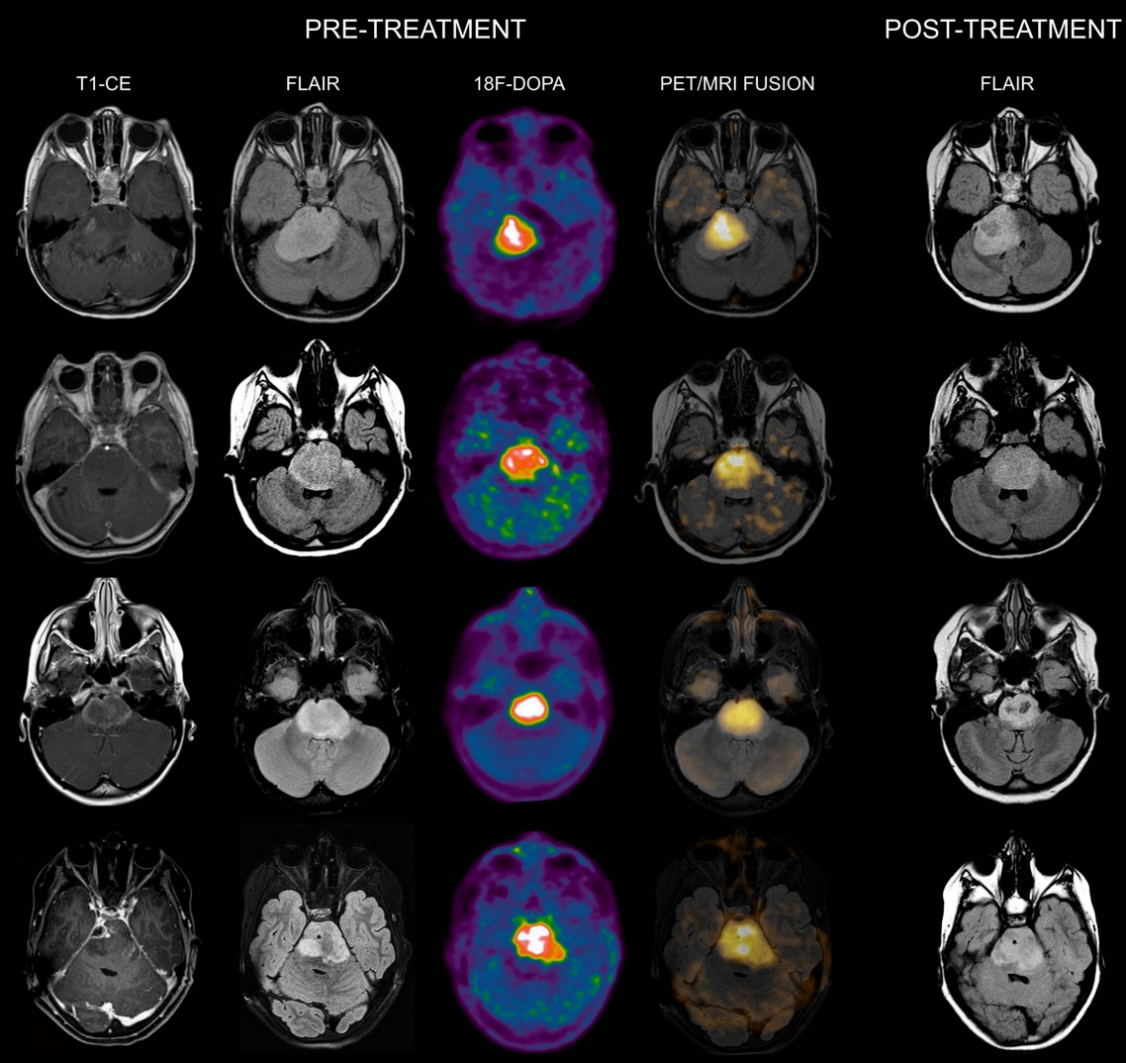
Co-registered and fused MRI and ^18^F-DOPA PET images of DIPGs with T/S > 1. First row: 4-year-old female. Pre-treatment contrast-enhanced (CE) T1-weighted imaging showed ring enhancement. MRI tumor volume was 40 cc. ^18^F-DOPA PET and fused ^18^F-DOPA PET/MRI revealed markedly increased uptake (T/S 1.09) with a PET tumor volume of 21 cc, corresponding to a uniformity of 52.5%. Post-treatment FLAIR demonstrated an MRI tumor volume of 28.9 cc. Overall survival (OS) was 9 months. Notice the coincidence between the tumoral component with increased uptake and the residual lesion, and the tumor volume reduction in the left ventrolateral pons corresponding to absence of increased uptake. Second row: 7-year-old male (anaplastic astrocytoma, H3K27M-mutant). Pre-treatment CE T1-weighted imaging did not reveal areas of ring enhancement. MRI tumor volume was 34.06 cc. On ^18^F-DOPA PET and fused ^18^F-DOPA PET/MRI the T/S was 1.27, the tumor volume 25 cc, and the uniformity 73.39%. Following treatment, the MRI tumor volume was of 33.7 cc. OS was 10 months. Third row: 10-year-old female. On pre-treatment CE T1-weighted imaging there was extensive ring enhancement. MRI tumor volume was 26 cc. On ^18^F-DOPA PET and fused ^18^F-DOPA PET/MRI the lesion presented a T/S of 1.7 and a tumor volume of 18 cc, corresponding to a uniformity of 69.23%. Post-treatment FLAIR image demonstrated an MRI tumor volume of 20 cc. OS was 6 months. Fourth row: 10-year-old male (glioblastoma, H3K27M-mutant). Pre-treatment CE T1-weighted imaging revealed a right focal area of ring enhancement. MRI tumor volume was 46 cc. ^18^F-DOPA PET and fused ^18^F-DOPA PET/MRI demonstrated markedly increased uptake (T/S 2.32) and a tumor volume of 31 cc, corresponding to a uniformity of 67.39%. Following treatment, the MRI tumor volume was 42 cc. OS was 6 months.
